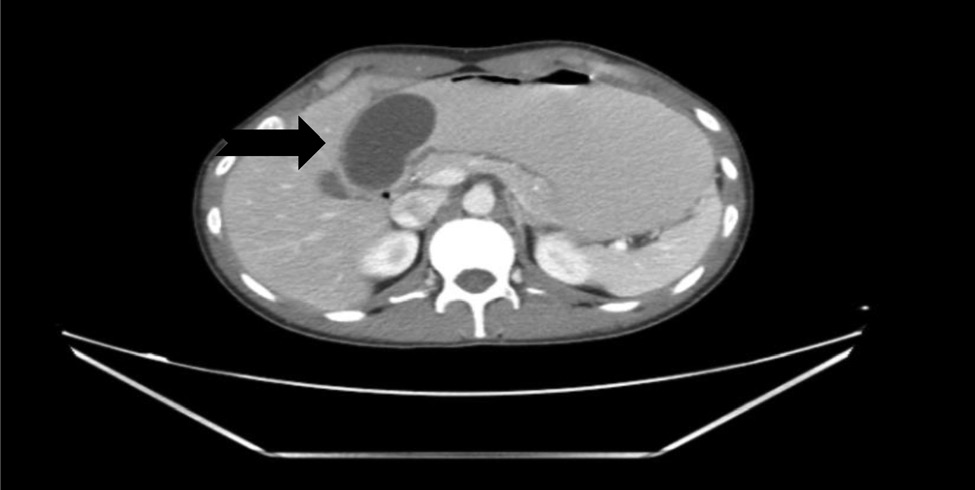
Computed tomographic scan showing 6.4 × 3.2 cm cystic structure in the distal stomach antrum adjacent to the pylorus producing partial gastric outlet obstruction.
